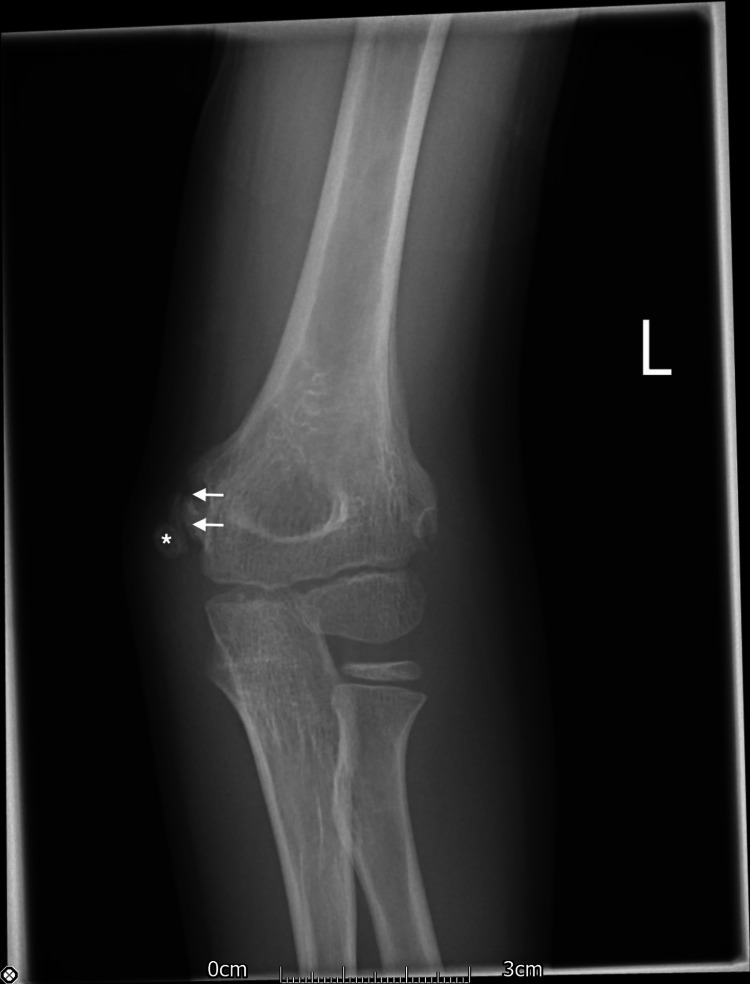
Six-month postoperative anteroposterior radiograph demonstrating osseous bridging of the medial epicondyle (asterisk). There is impression of an osseous tunnel formed along the medial epicondyle (arrows). The patient had persistent neurological symptoms and paraesthesia in the median nerve distribution.
